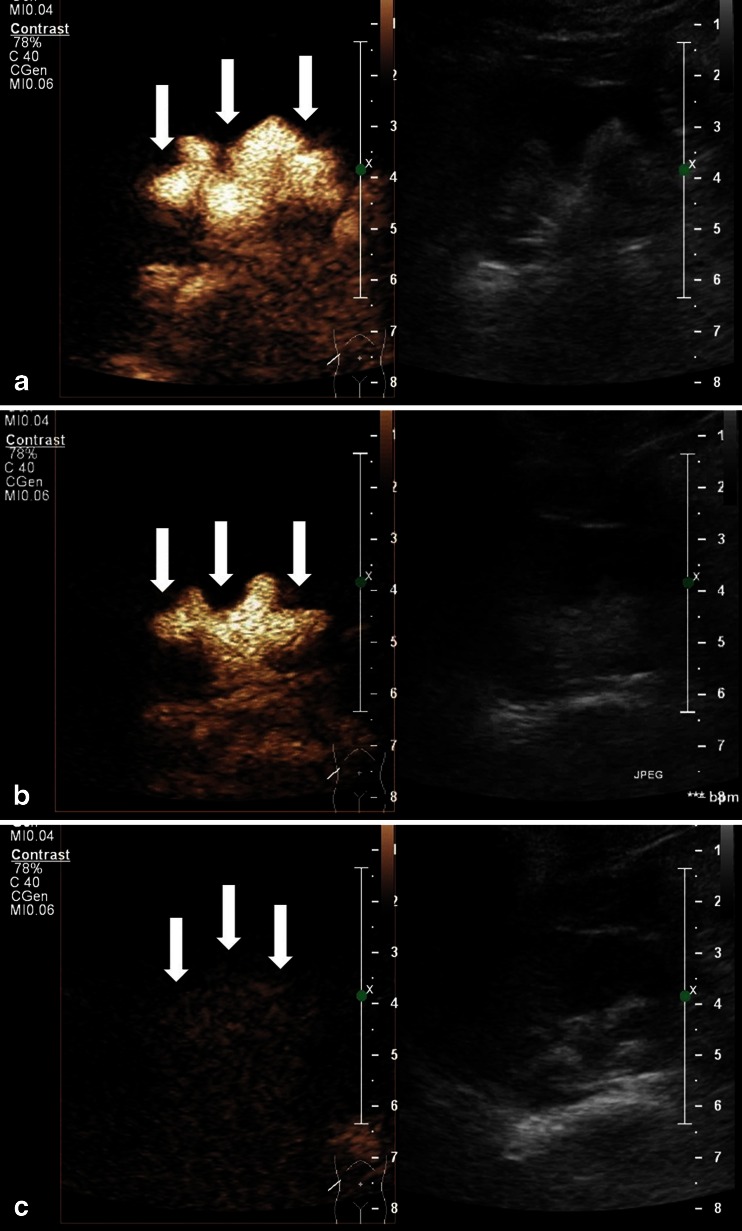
Intraoperative contrast-enhanced urosonography (ce-US). Two-dimensional US in contrast imaging side-to-side mode (contrast image on left side, fundamental mode on right side). a Ce-US performed before endoscopic treatment shows right-side low-pressure (passive) VUR grade IV (*arrows*). b Ce-US performed after the first injection of bulking material shows downgrading of the right-side VUR to grade III (*arrows*). c Ce-US performed after the second injection of bulking material shows no VUR on the right side (*arrows*)
